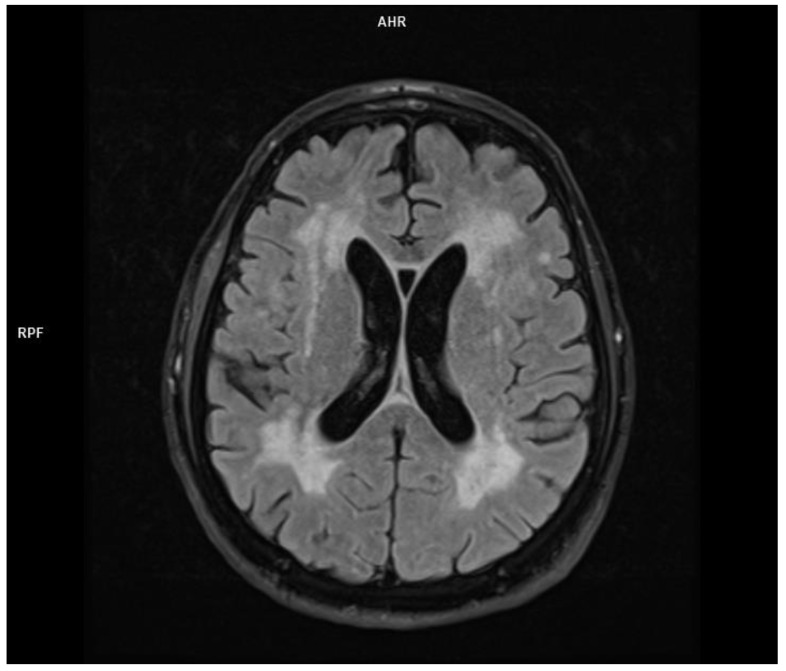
Moderate cortical atrophy and supratentorial ventricular system dilatation—Flair sequence (MRI imaging).
